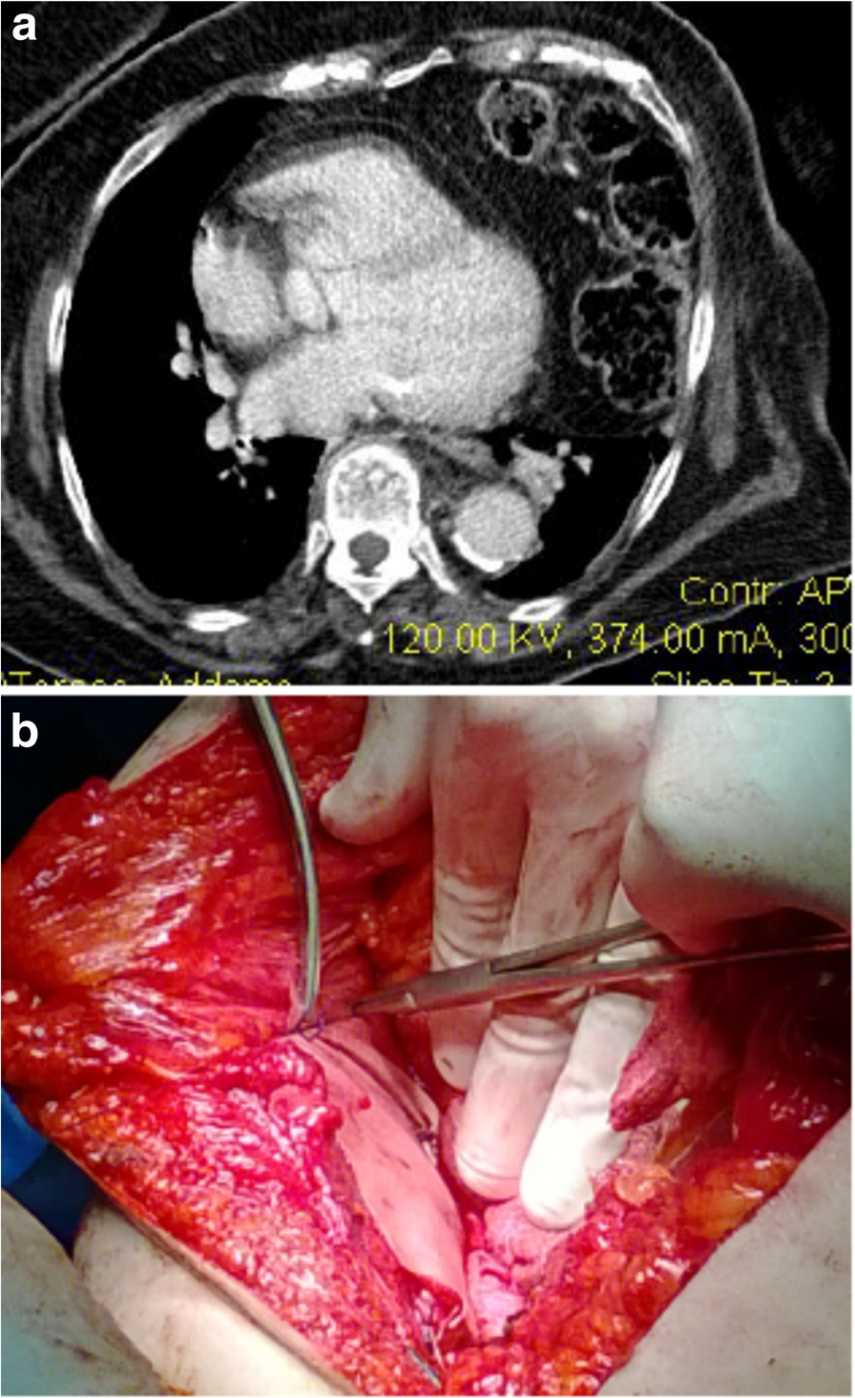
a CT scan suggests *left* diaphragmatic hernia which allowed migration of colon in *left* chest. b Intraoperatively, biological mesh repairing defect
